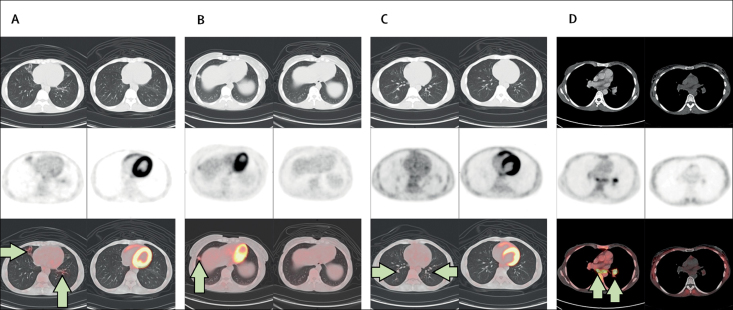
PET-CT images from four patients investigated for tuberculosis Four patients who were face-mask-positive but sputum-negative for *Mycobacterium tuberculosis* at screening were followed up for 20 weeks. Matched 6-week (left) and 20-week (right) images for each patient (A–D). CT images are shown in the upper windows, PET scans in the middle windows, and the fused dataset in the lower windows. (A–C) Arrows show parenchymal lung changes (tree and bud) with associated increased ^18^F-fluorodeoxyglucose uptake on the 6-week scan, which resolved completely at 20 weeks. (D) Arrows show mediastinal and left hilar lymph nodes, which were active on the 6-week scan and resolved completely at 20 weeks.
